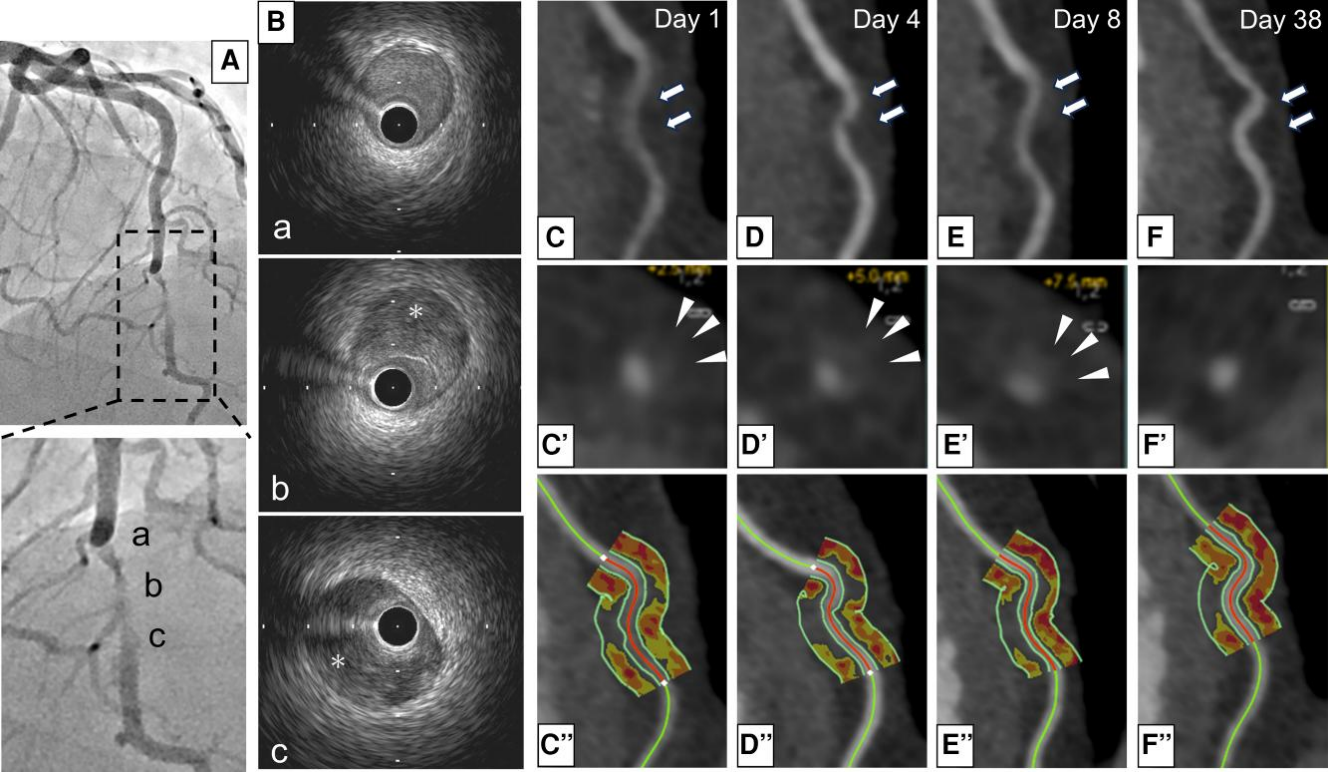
A 53-year-old woman was hospitalized due to ST-elevation myocardial infarction. Emergent coronary angiography revealed a severe stenotic lesion in her left anterior descending artery (*Panel A* and see Supplementary material online, *Video 1*). Intravascular ultrasound imaging revealed a narrowed true lumen compressed by intramural haematoma (Supplementary material online, *Video 2* and *Panel B*, a–c). She was diagnosed with spontaneous coronary artery dissection (SCAD). Following the commencement of medical therapies (aspirin 100 mg, bisoprolol 1.25 mg), this lesion was serially evaluated by coronary computed tomography angiography. At Day 1, diffuse lumen narrowing and wall thickening were observed. Furthermore, this lesion exhibited a high pericoronary adipose tissue (PCAT) attenuation [−73 Hounsfield unit (HU)], suggesting an elevated coronary inflammatory activity (*Panel C*). These features still continued to exist at Days 4 (−75 HU) and 8 (−78 HU) (*Panels D* and *E*). However, at Day 38, in addition to the disappearance of diffuse narrowing and wall thickening, PCAT attenuation improved (−91 HU) (*Panel F*).
